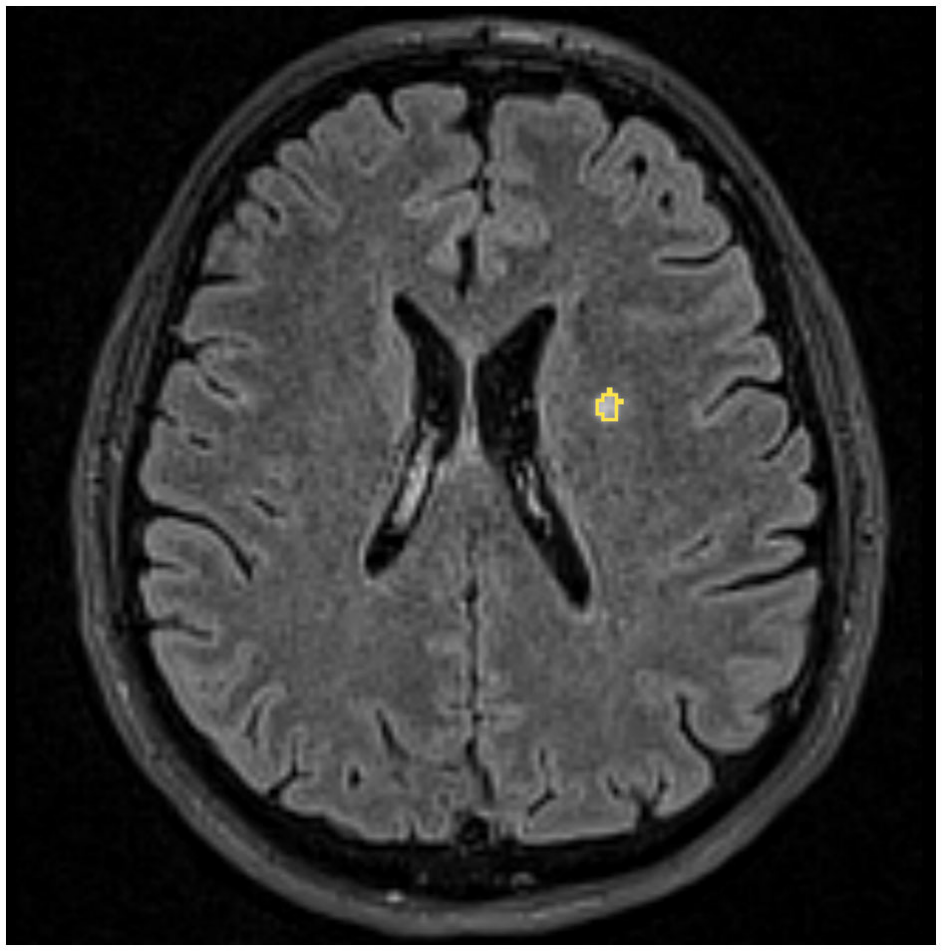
Delineation of a white matter lesion in a female migraineur. An example of an axial FLAIR image used to assess white matter lesion is shown in the background. Yellow indicates the outline of a manually delineated white matter lesion.
